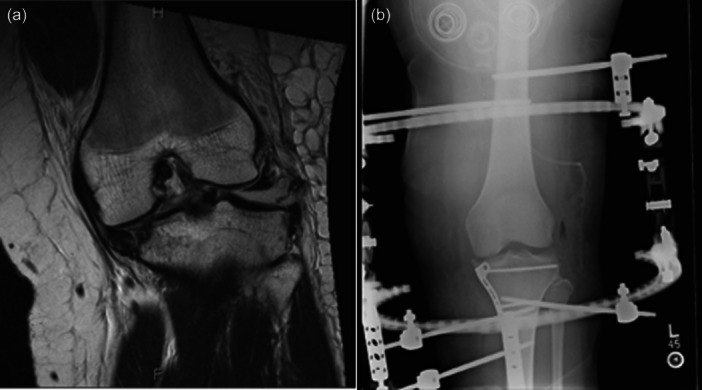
(a) Coronal proton density‐weighted MRI image demonstrating lateral coronal tibiofemoral subluxation, lateral compartment distraction and peripheral rim medial tibial plateau fracture, not shown is the associated posterolateral corner disruption with avulsion of the lateral collateral ligament, biceps femoris and patellofemoral ligament from the fibular head. (b) Post reduction X‐ray with internal fixation of tibial plateau fracture and application of a Compass Hinge External Fixator. MRI, magnetic resonance imaging.
